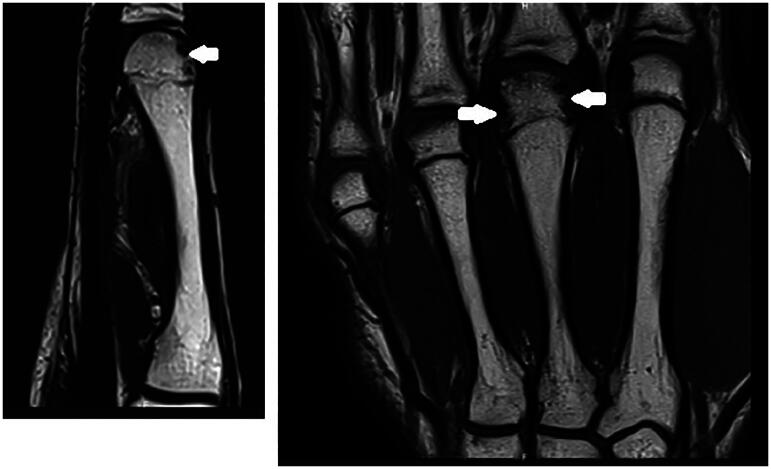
MRI April 2023. Normalization of the abnormalities, in T1 weighted images.
